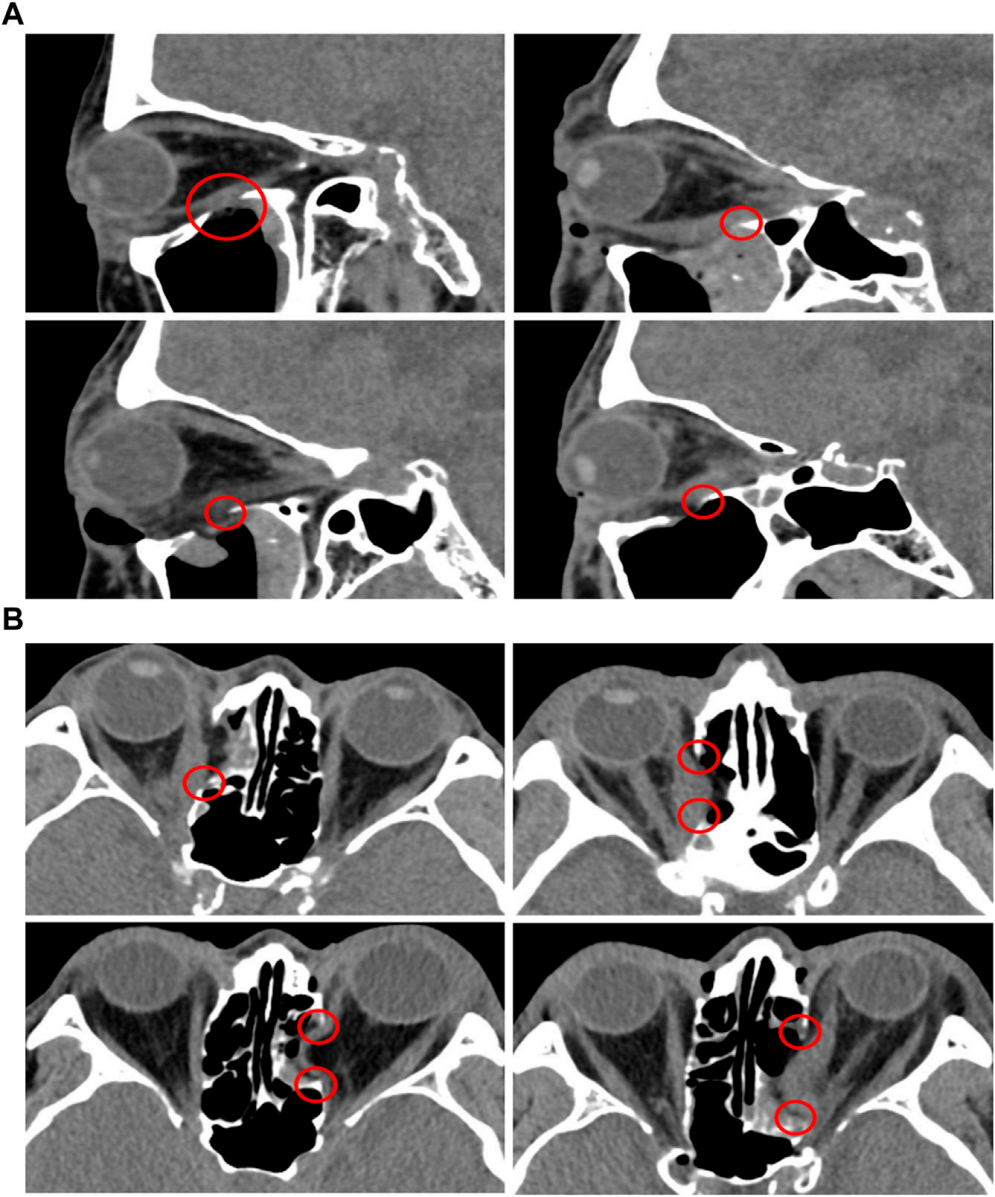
CT analysis of TED patients who underwent orbital decompression surgeries. (A) Sagittal plane. (B) Axial plane.
